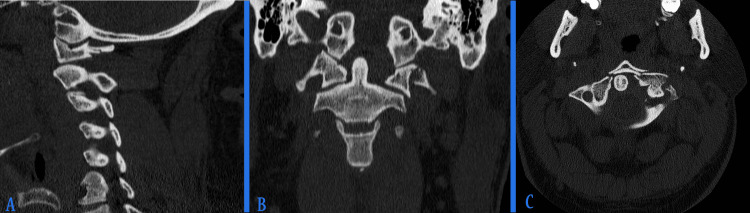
Computed tomography scan shows C1 Jefferson burst fracture extending to the left transverse foramen with atlantoaxial subluxation to the right side: (A) sagittal cut, (B) coronal cut, and (C) axial cut.
